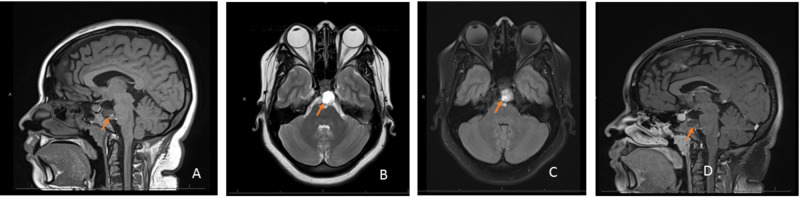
Brain MRI with and without contrast Brain MRI with and without contrast performed on admission. A - sagittal T1-weighted view: the mass appears heterogeneously hypointense (arrow); B - axial T2-weighted view: the mass appears homogenously hyperintense (arrow); C - axial FLAIR: the mass has slightly heterogeneously hyperintense diffusion (arrow); D - gadolinium-enhanced sagittal T1-weighted view: a lesion in the retroclival prepontine location with no contrast enhancement (arrow) MRI: magnetic resonance imaging; FLAIR: fluid attenuation inversion recovery
